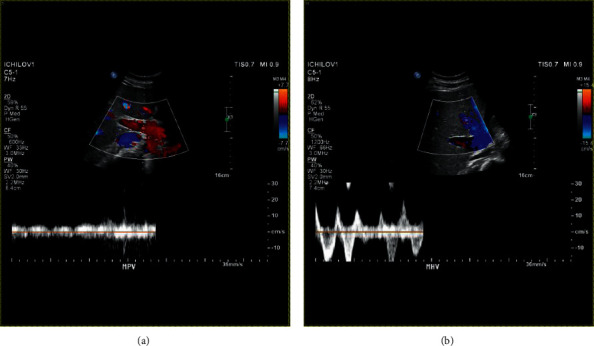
Liver Doppler sonography showing normal venous flow in the main portal vein (a) and three hepatic veins (b).
